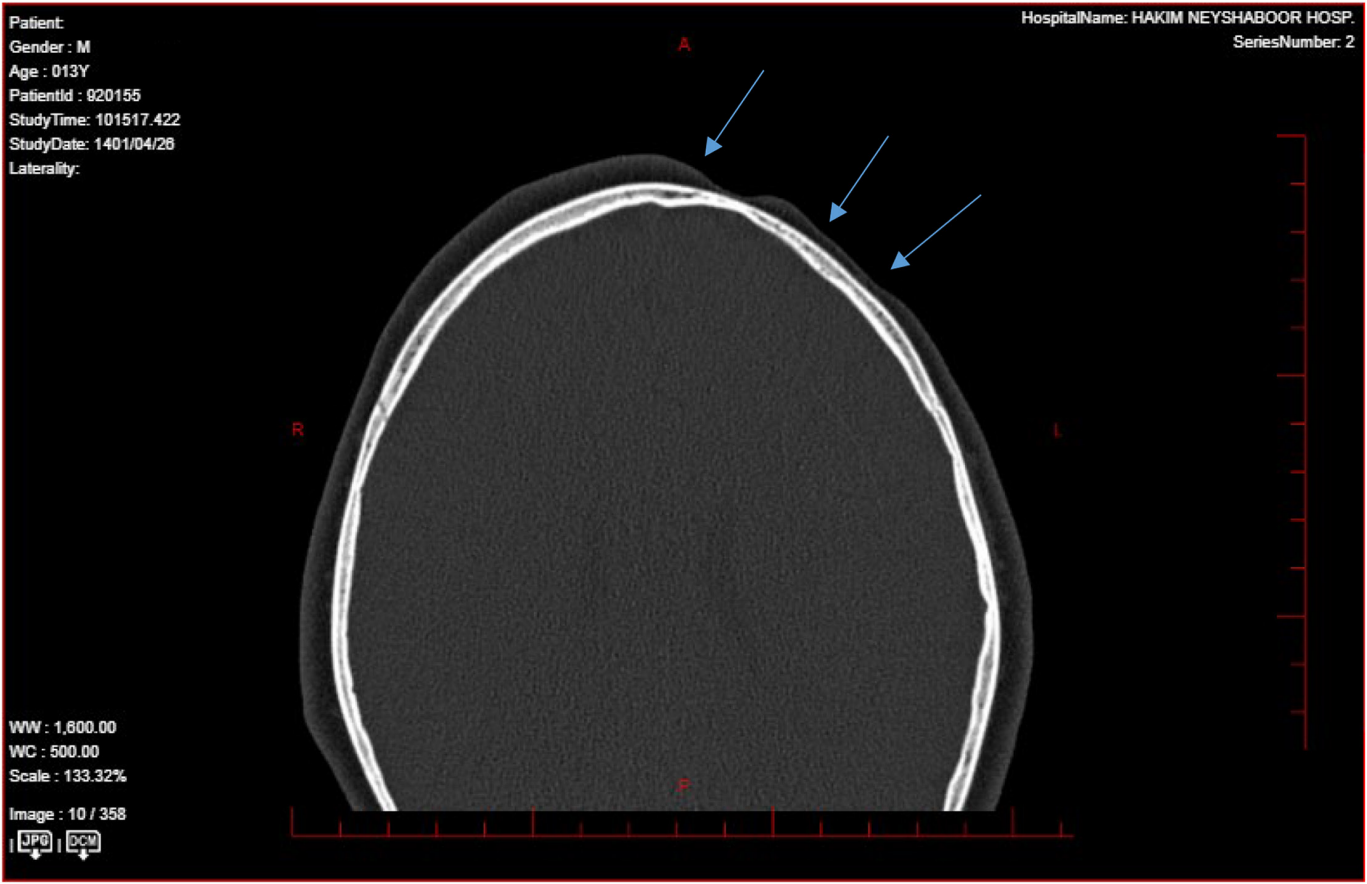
CT scan image shows that the bone tissue is not involved and only the subcutaneous tissue is atrophied.
